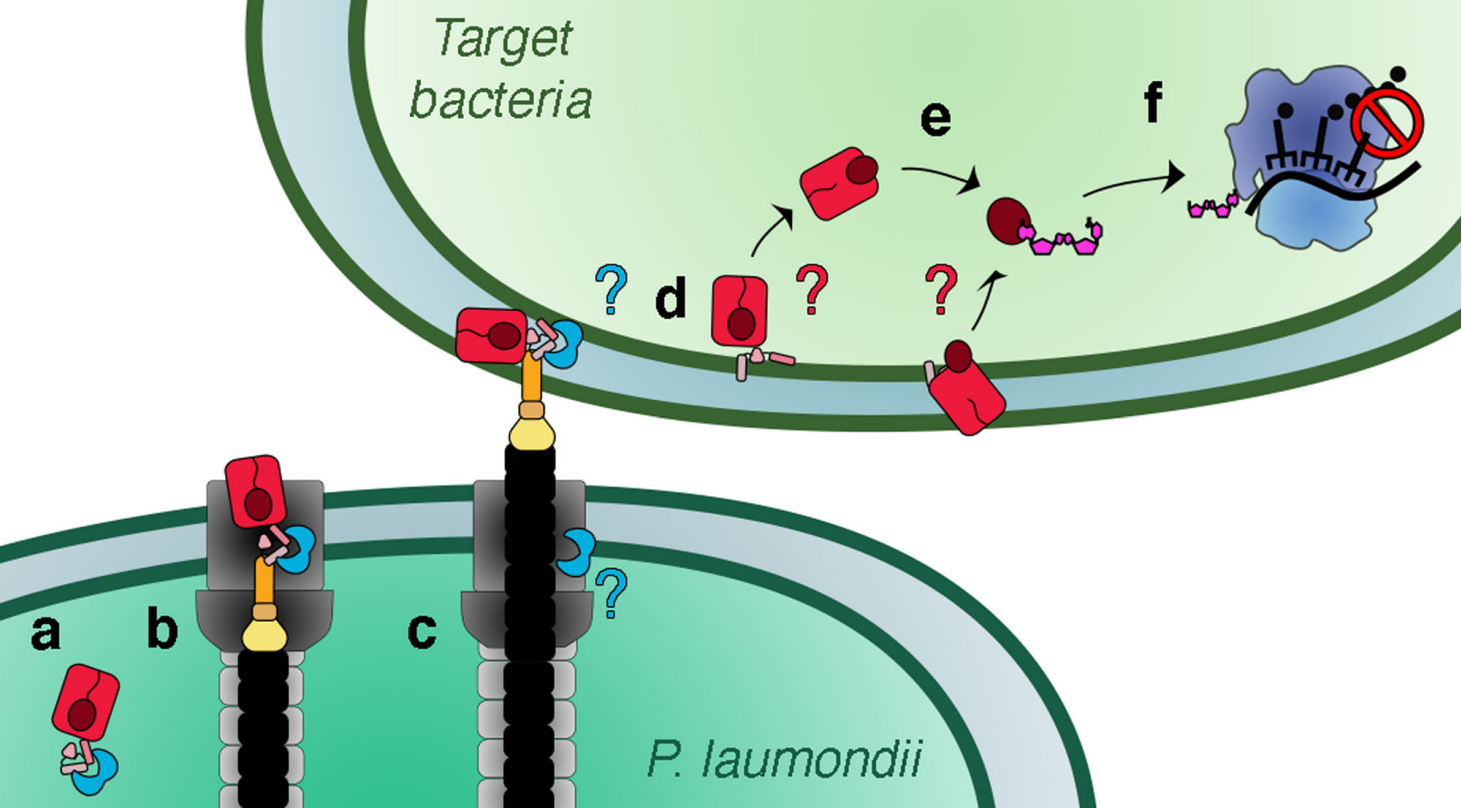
Model for Rhs loading and delivery by the type VI secretion system. a The EagR chaperone (blue) binds to the Rhs^NT^ hydrophobic domains to stabilize the Rhs protein (red) prior loading on the T6SS. b The Rhs protein is loaded on the C-terminus of the VgrG spike (orange) via its N-terminal prePAAR-PAAR domain. c Sheath contraction propels the inner tube (black) to inject the VgrG loaded with Rhs into the target cell. It is not known whether the EagR chaperone remains in the attacker cell or whether it is translocated together with the VgrG-Rhs complex. d The Rhs1 protein is directly delivered in the cytoplasm, or if delivered in the periplasm, the Rhs^NT^ hydrophobic transmembrane domains insert into the inner membrane of the target cell and are used to translocate the Rhs β-barrel and/or the toxic domain into the cytoplasm of the target cell. Alternatively, the Rhs β-barrel itself inserts into the membrane. e N- and C-terminal autoproteolysis opens the Rhs shell and releases the Rhs^CT^ toxin into the target cell cytoplasm. f The *P. laumondii* Rhs1 C-terminal toxin domain, Tre23, arrests translation by ADP-ribosylation of the 23 S rRNA.
